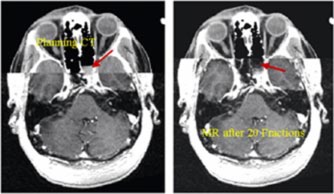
A sample of MR image obtained after 20 out of 35 treatments is fused with the planning CT image. The tumor is seen on the planning CT image, but is almost invisible on the MR image as shown by the red arrow.
